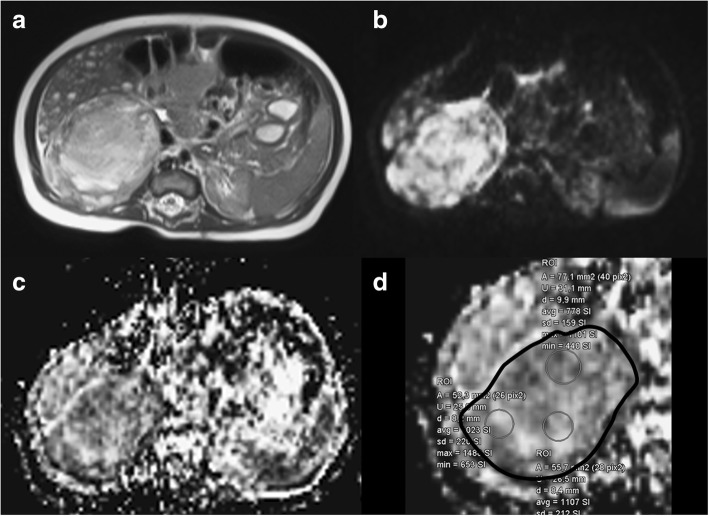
Baseline MRI of a 16-month-old boy with neuroblastoma of the left adrenal gland and suspected liver metastases (liver lesions not shown). The tumour signal is notably more heterogeneous than that of the lesion shown in Fig. 2 and comprises some high-signal areas on the T2-weighted image (a). In b, a high signal on DWI (*b* = 800 s/mm^2^) can be appreciated while a low signal is visible on the ADC map (c) is, however, predominant within the tumour. The presented cross-sectional image was chosen for analysis, as the cross section at the largest tumour diameter included a higher proportion of heterogeneous tumour signal. The black solid line overlay on the magnified ADC map highlights the large ROI drawn for study purposes (d). Mean baseline ADC was measured as 0.706 × 10^−3^ mm^2^/s in this eventually relapsing tumour. Three small ROIs yield ADC values of 0.589, 0.638, and 2.841 × 10^−3^ mm^2^/s, the latter equalling liquefied tumour necrosis
